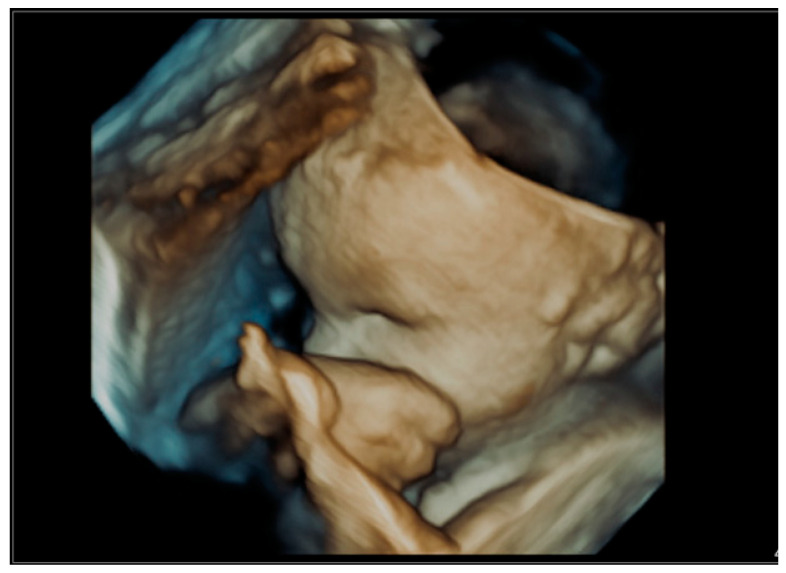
An image of a fetal ear auricle in 3D ultrasound examination at the gestational age of 35 weeks and 1 day. A genetic test of the amniotic fluid: the use of whole genome oligonucleotide microarrays (CytoSure Constitutional v3 (8x60k), Oxford Gene Technology, GRCh37/hg19) with an average resolution of 120 kpz–arr(X,Y)x1,(1-22)x2–No abnormalities were detected.
